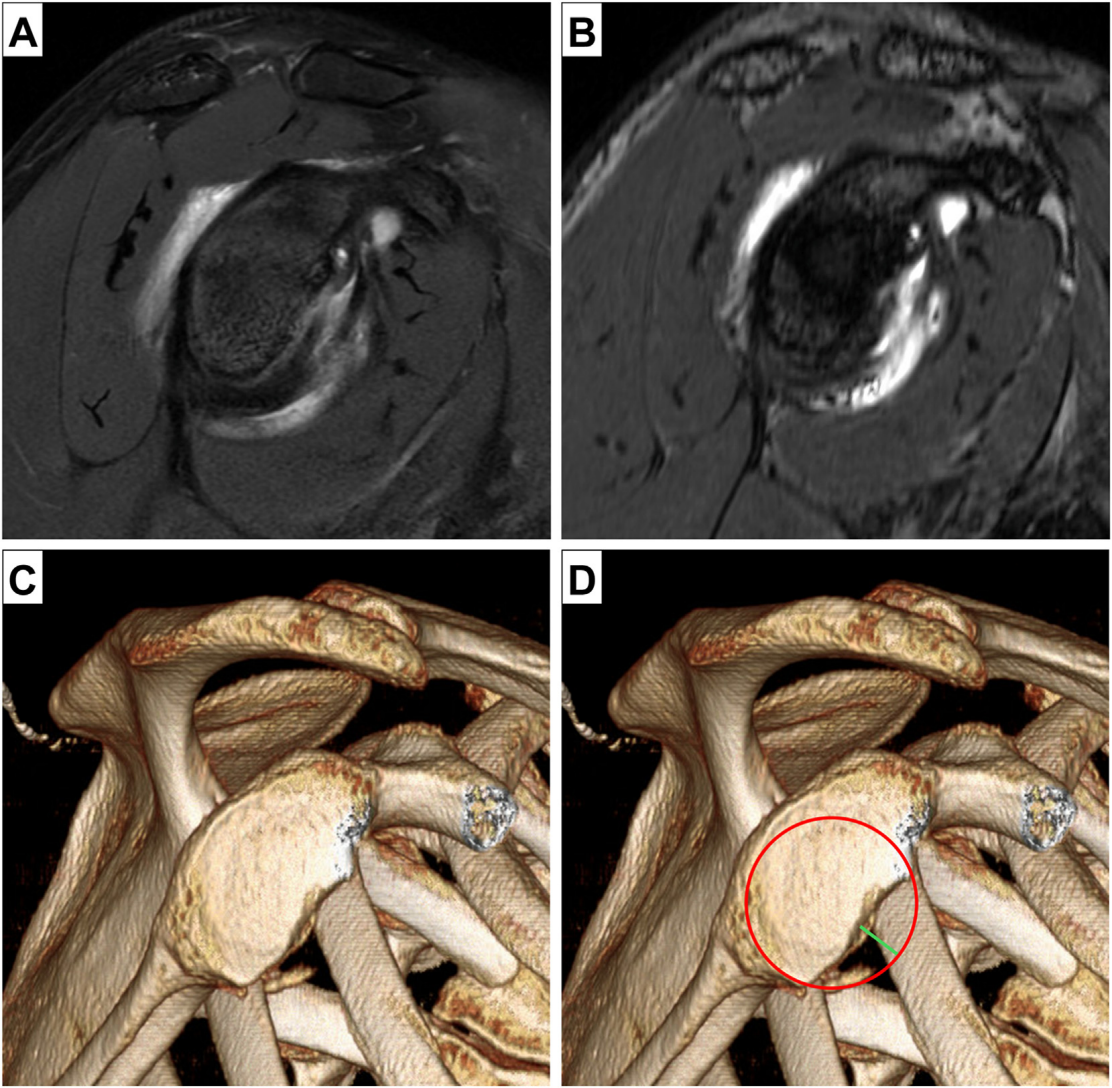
En face sagittal views of a patient with approximately 17% glenoid bone loss after multiplanar reformation on (A) PD FS MRI, (B) FIESTA MRI, and (C) 3D CT reconstruction. (D) Best-fit circle linear method for measurement of native glenoid diameter and linear defect to calculate glenoid bone loss (% GBL = defect width/glenoid diameter × 100). The *red circle* matches the contour of the posteroinferior glenoid and the *green line* corresponds to the defect width. This method was performed on all 3 imaging modalities. *MRI*, magnetic resonance imaging; *PD FS*, proton density fat-saturation; *FIESTA*, fast-imaging employing steady-state acquisition; *GBL*, glenoid bone loss; *3D*, three-dimensional; *CT*, computed tomography.
